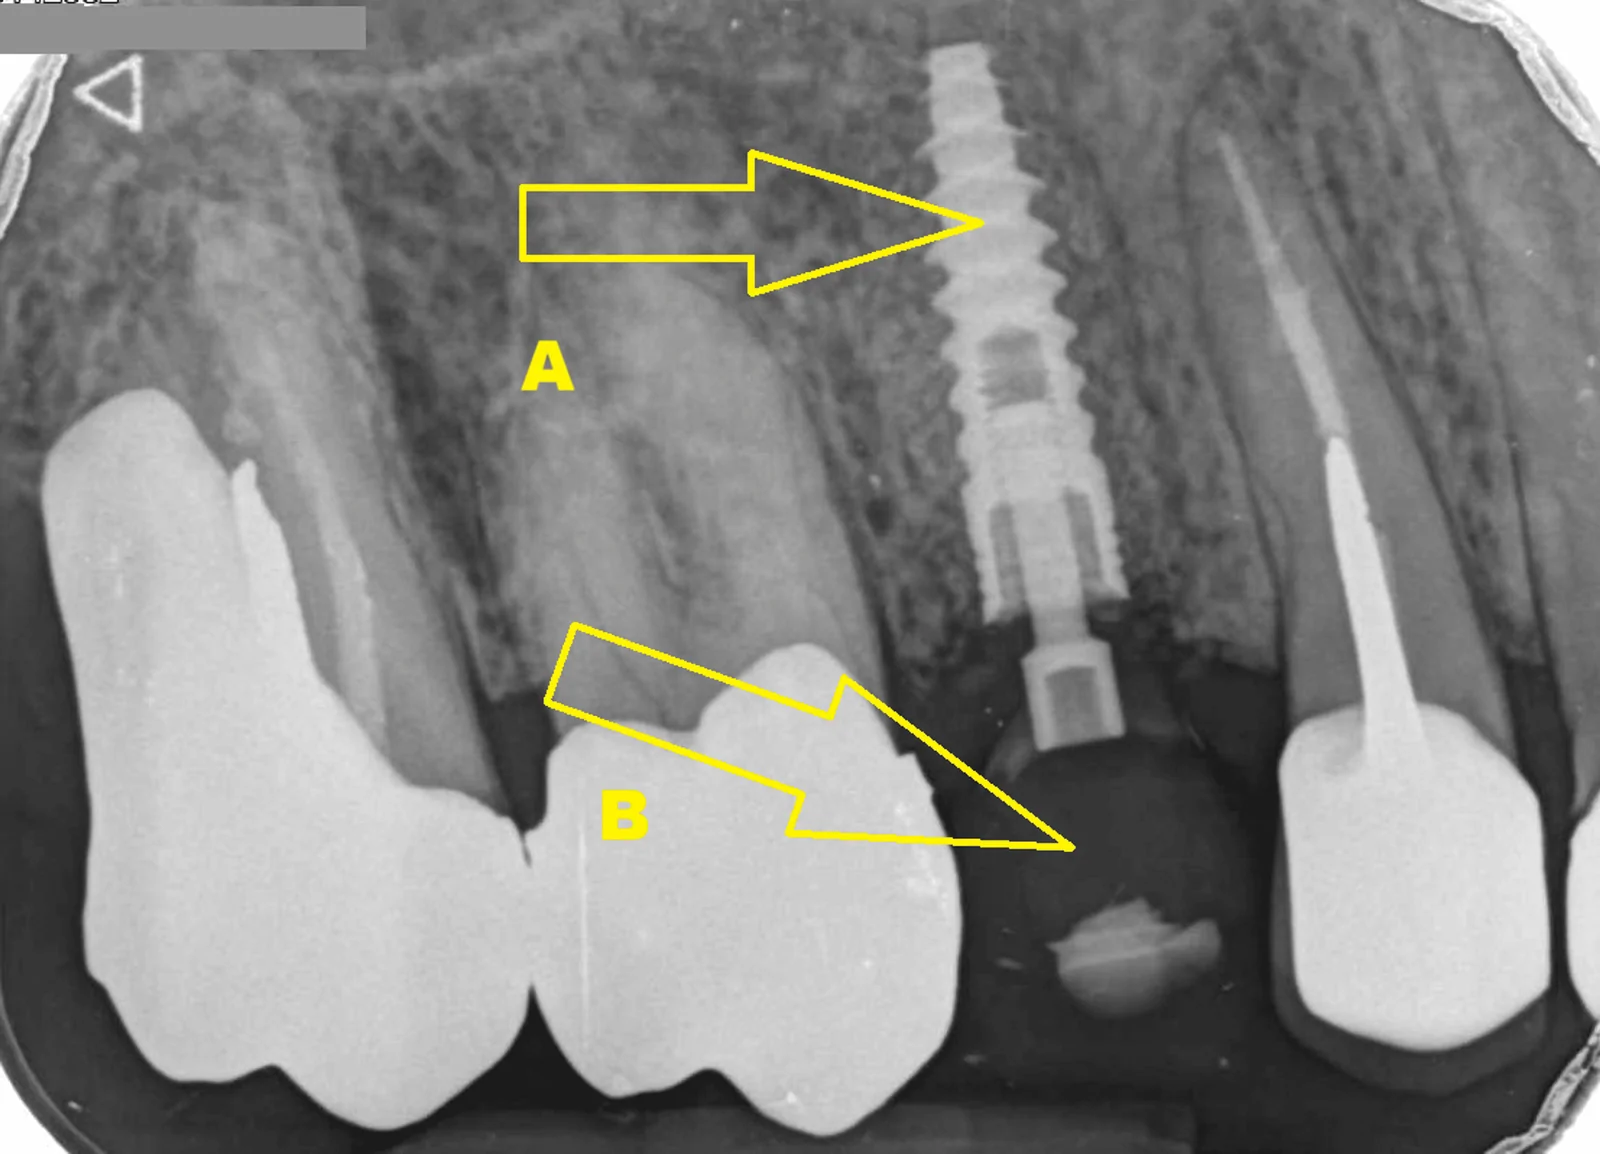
Postoperative radiograph following implant placement and provisional restoration of the maxillary right second premolar (tooth #4). Postoperative periapical radiograph obtained six months after alveolar ridge preservation, demonstrating successful implant placement in the maxillary right second premolar (tooth #4) site. Arrow A indicates the dental implant in its final position. Arrow B indicates the provisional (temporary) crown, which was placed during the provisional restorative phase before fabrication of the definitive implant-supported restoration.
